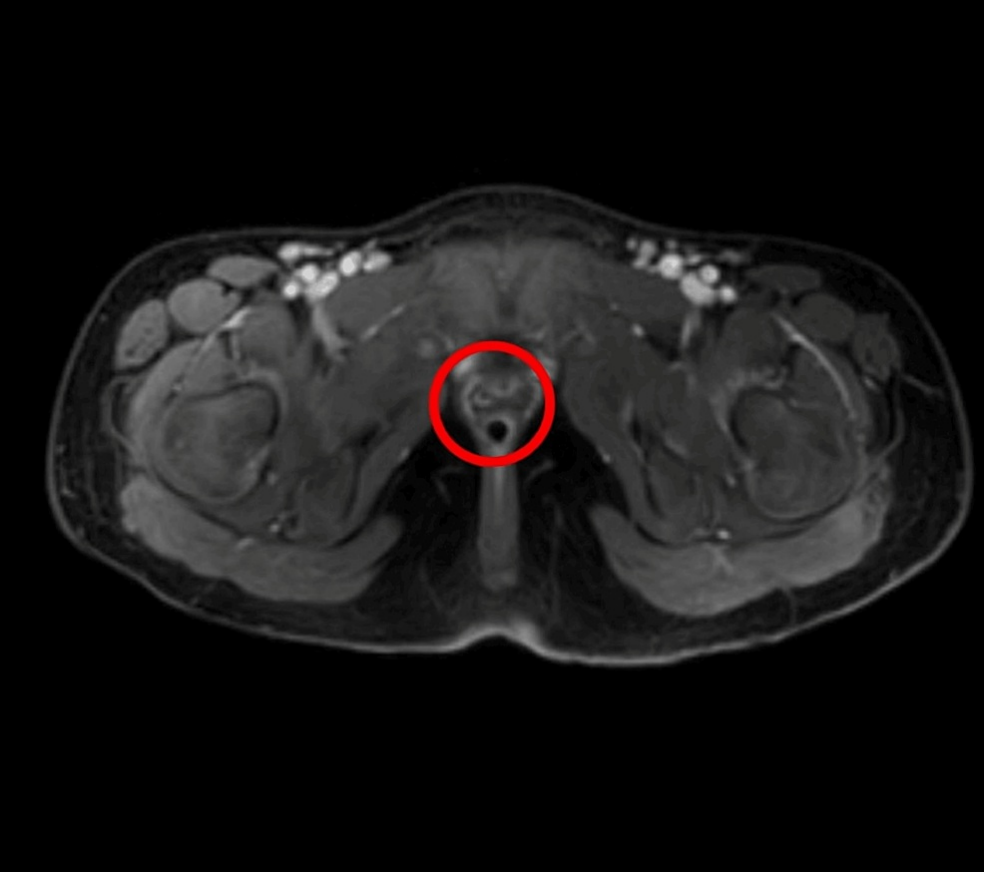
MRI showing bilateral ectopic ureters
